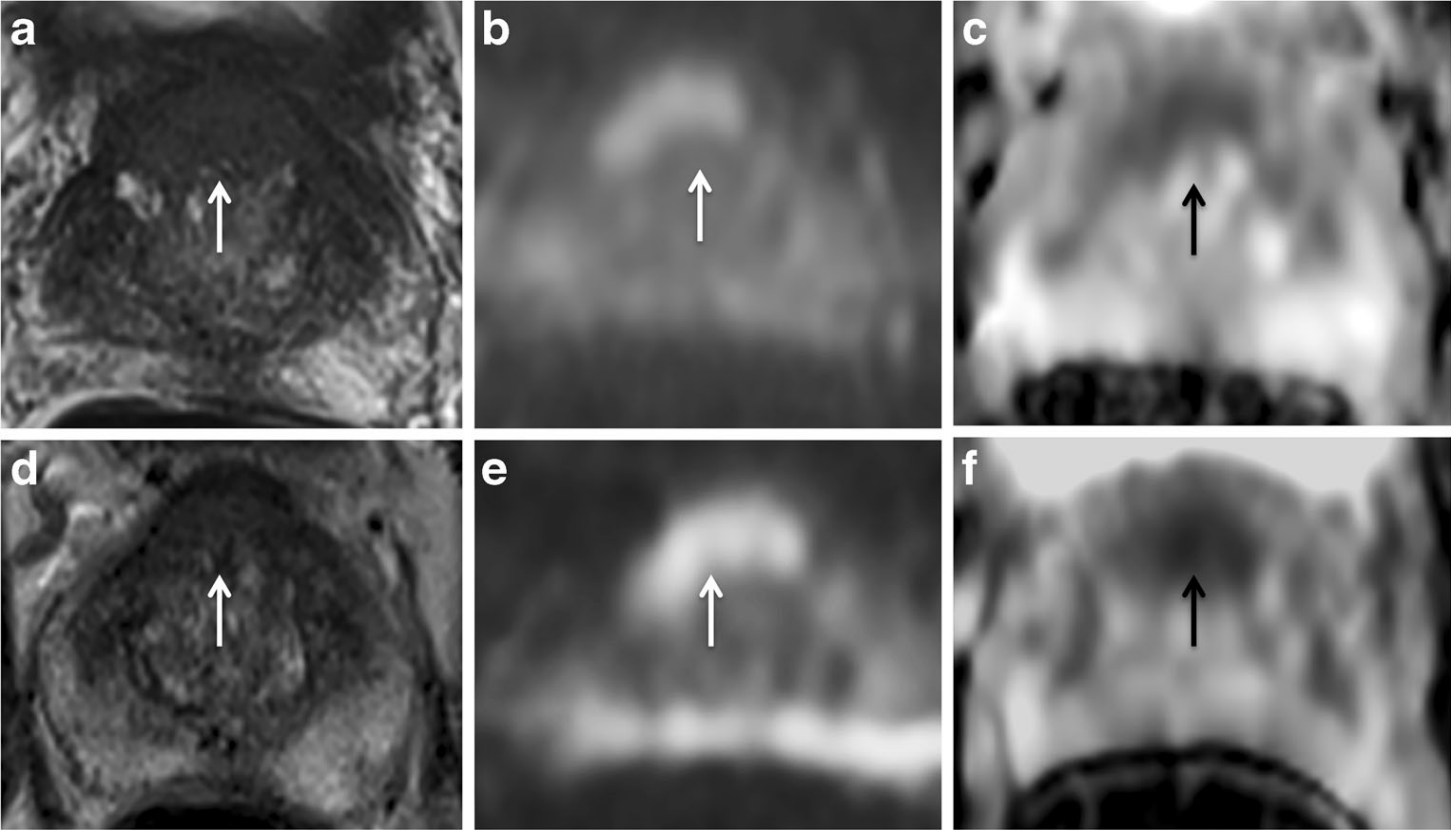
67-year-old man with a presenting PSA of 6.64 ng/mL and Gleason Score 3 + 3. At baseline MRI, the pathological area of decreased signal intensity in the anterior part of the transition zone (arrow) on the axial T2-weighted image (A) corresponds to the high-signal intensity on the DWI image (B) and low-signal intensity on the ADC map (C), with a reduced ADC value (0.87 x 10^-3^ mm^2^/s). At 6-month MRI (PSA: 8.10 ng/mL) the pathological area is still recognisable (arrow) in all the three scans (D,E,F) and a decrease in the mean ADC value was observed (0.63 x 10^-3^ mm^2^/s). There was an increase in conspicuity of 36% on the ADC map. This patient was in the placebo arm
